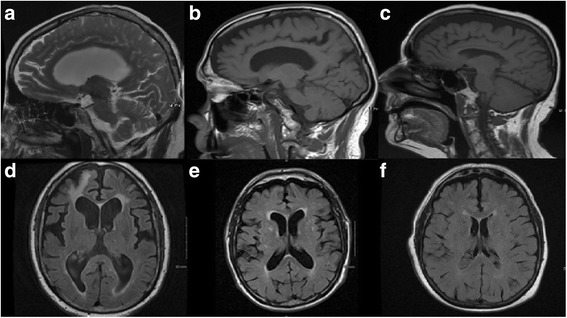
Brain MRI of Case #1–3. Sagittal T2-weighted (*upper row*) and axial FLAIR (lower *row*) brain MRIs. Patient 1 (a and d) showed mild to moderate atrophy in the frontotemporal regions, minimally asymmetric, with right frontal encephalomalacia. Patient 2 (b and e) showed similar findings with somewhat lower burden of associated periventricular and spotty subcortical *white* matter increased signal. Patient 3 (c and f) showed mild diffuse atrophy with minimal periventricular and subcortical *white* matter disease
